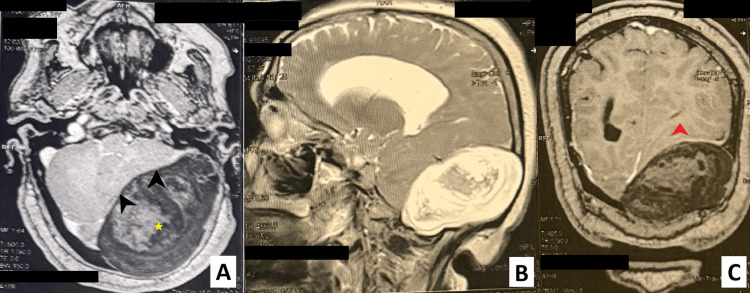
Preoperative MRI of the brain showing a massive posterior fossa lesion. A: Axial T1-weighted MRI with contrast enhancement showing a large extra-axial hypointense to isointense lesion (star) occupying the left posterior fossa, causing marked compression of the cerebellar hemisphere and brainstem (black arrow head). The lesion shows no contrast uptake after gadolinium injection. B: Sagittal T2-weighted MRI demonstrating a giant heterogeneously hyperintense mass with lobulated contours, extending inferiorly and posteriorly, with significant mass effect on the posterior fossa structures. C: Coronal T1- weighted sequence with contrast enhancement showing mass effect on the left lateral ventricle (red arrow head) with no significant hydrocephalus.
